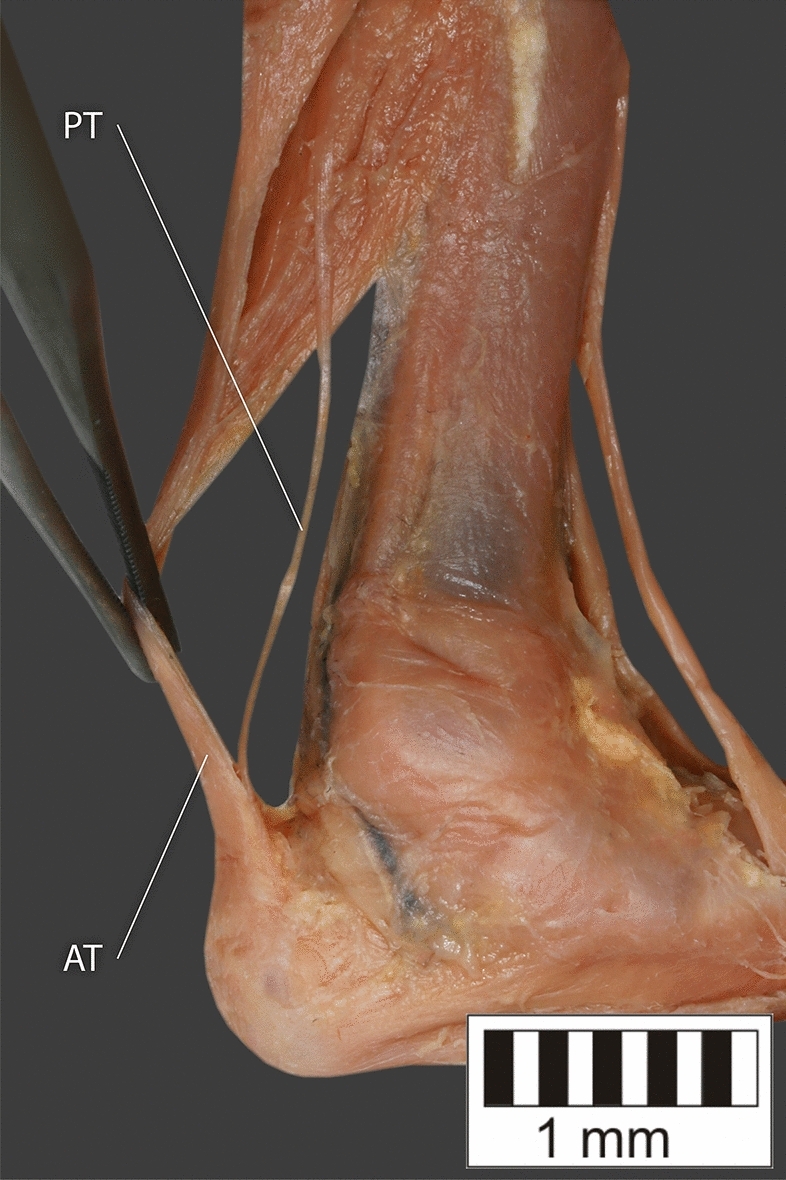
Type VII of plantaris tendon. *CT* calcaneal tuberostity, *AT* Achilles tendon, *PT* plantaris tendon.
